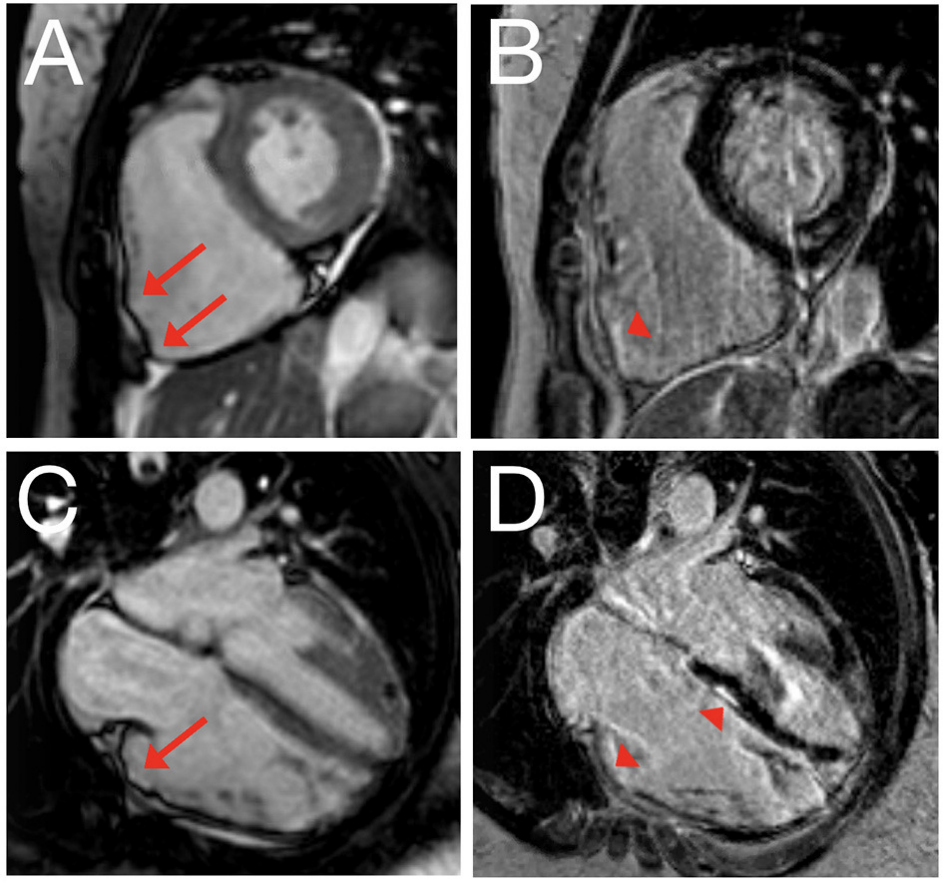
Cardiac magnetic resonance imaging of 22-year old female with ARVC. Still image of cine clip for short axis (A) and long axis (C) view, with corresponding delayed enhancement images obtained using phase-sensitive inversion recovery in short axis (B) and long axis (D) view. Red arrows denote dyskinetic segments with outward bulging in systole; arrowheads denote corresponding delayed enhancement.
